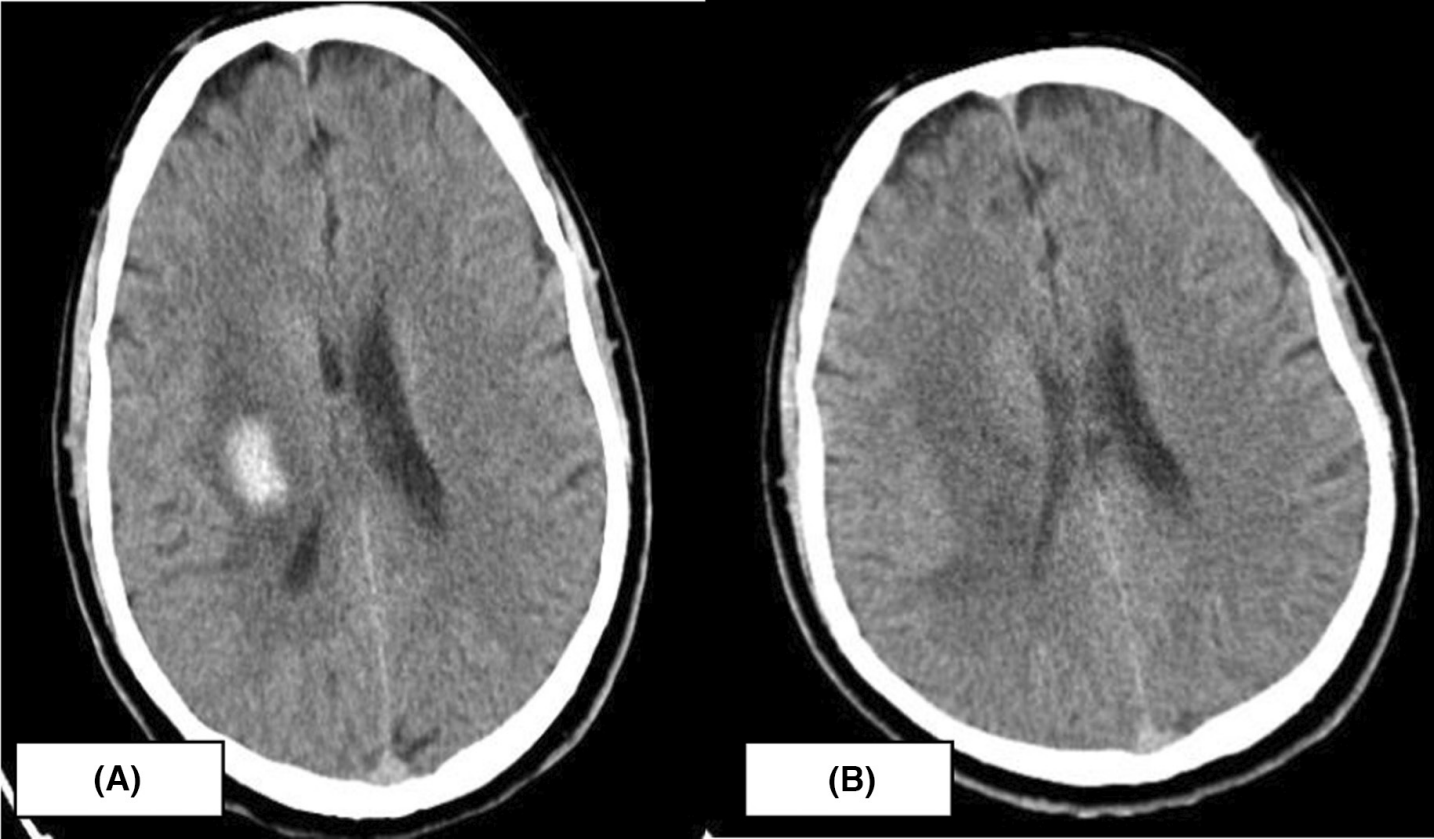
CT head showing significant resorption of the hematoma (12 × 24 mm) after 20 days since admission.
